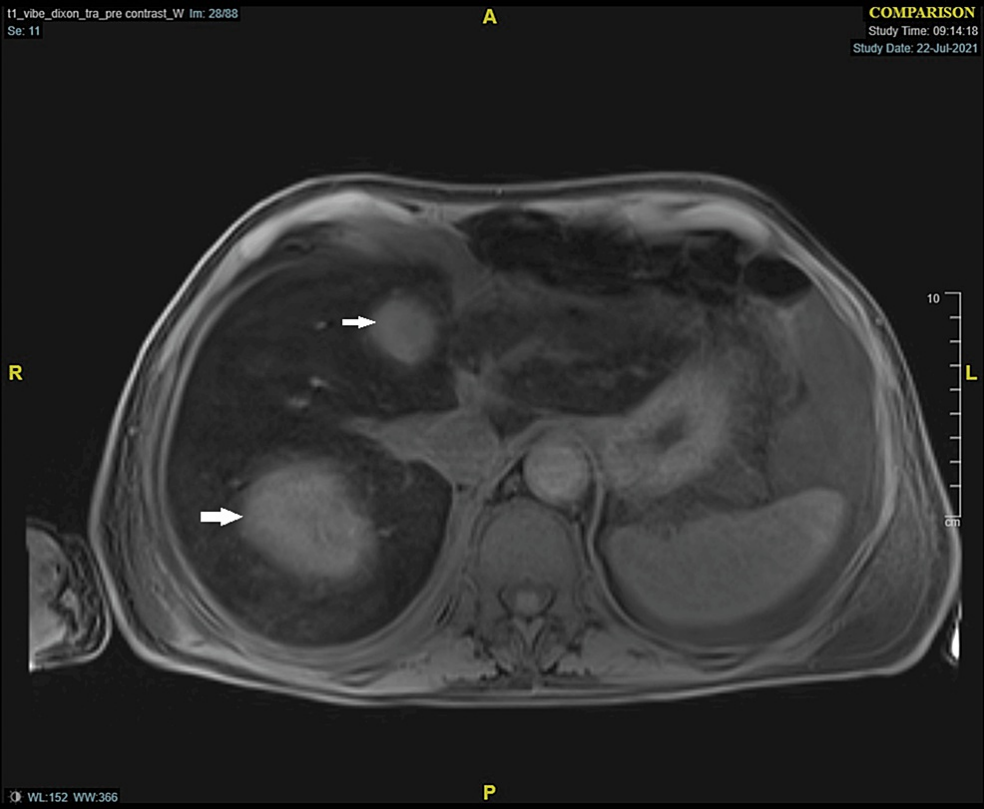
MRI liver Arrow: Liver abscess
